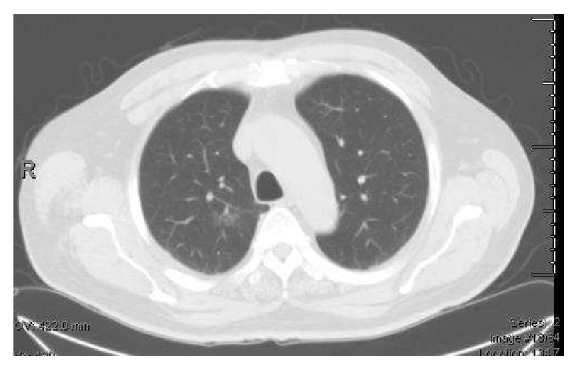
CT scan showing a 1.9 × 1.1 cm right upper lobe lung mass.
